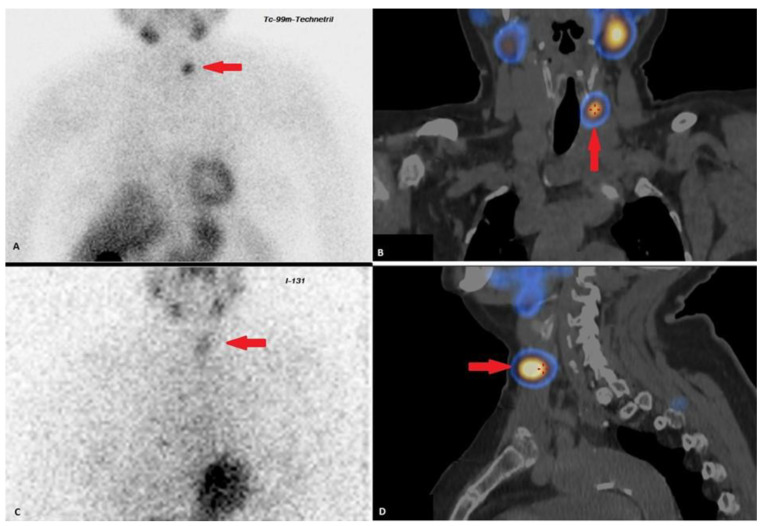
(A) planar scintigraphy with ^99^mTc-MIBI (←); (B) SPECT-CT frontal slice, high uptake of ^99^mTc-MIBI (↑); (C) Planar whole-body scintigraphy with I-131, (←) tissue accumulating 131I is visualized in the projection of the thyroid bed, on the left; (D) SPECT-CT sagittal slice, scanning was performed 90 min after the administration of 700Mbq of ^99^mTc-MIBI, (→) lesion in the left thyroid bed with high uptake of ^99^mTc-MIBI.
